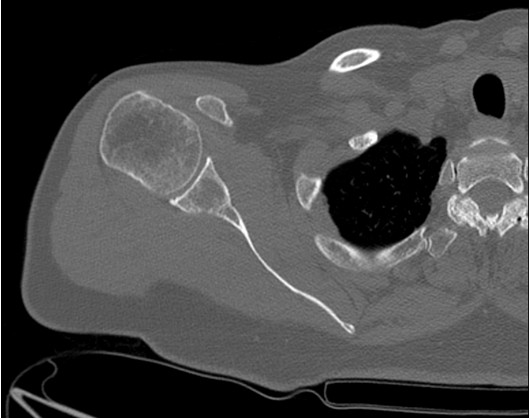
Magnetic resonance imaging scan of the patient’s right shoulder shows permeative destruction of the right scapula (black arrow).
